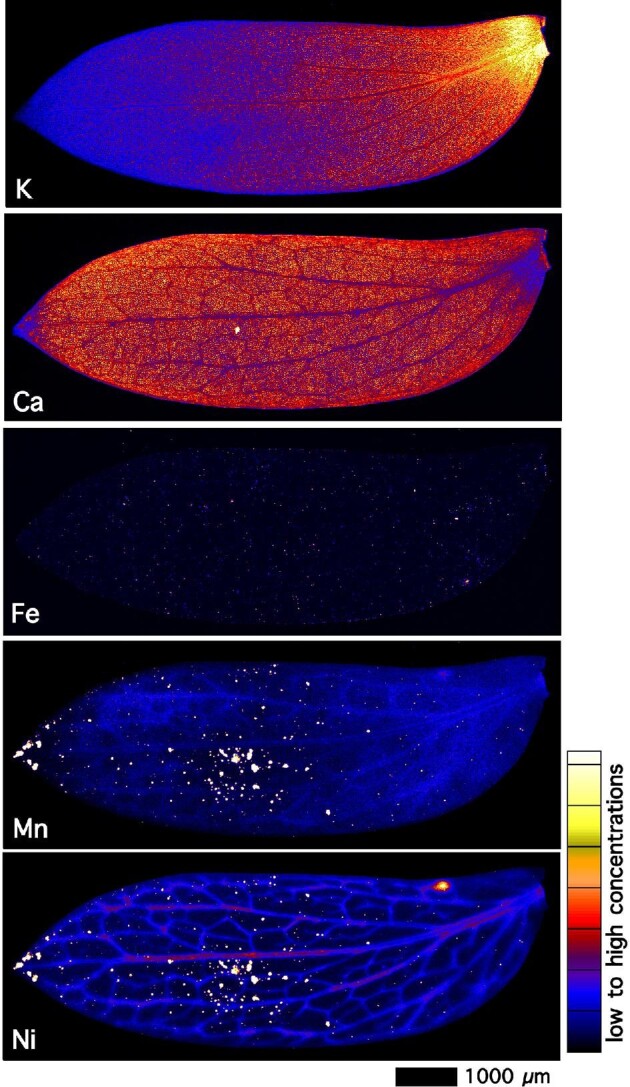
Elemental micro-X-ray florescence (μXRF) maps of whole freeze-dried *P. balgooyi* leaf showing K, Ca, Mn, and Ni distribution. The elemental image measuring 9.8 × 3.7 mm in area was acquired in an 8-μm step size with a 10 ms dwell per pixel. Acquired at the P06 beamline of the German Synchrotron (DESY).
